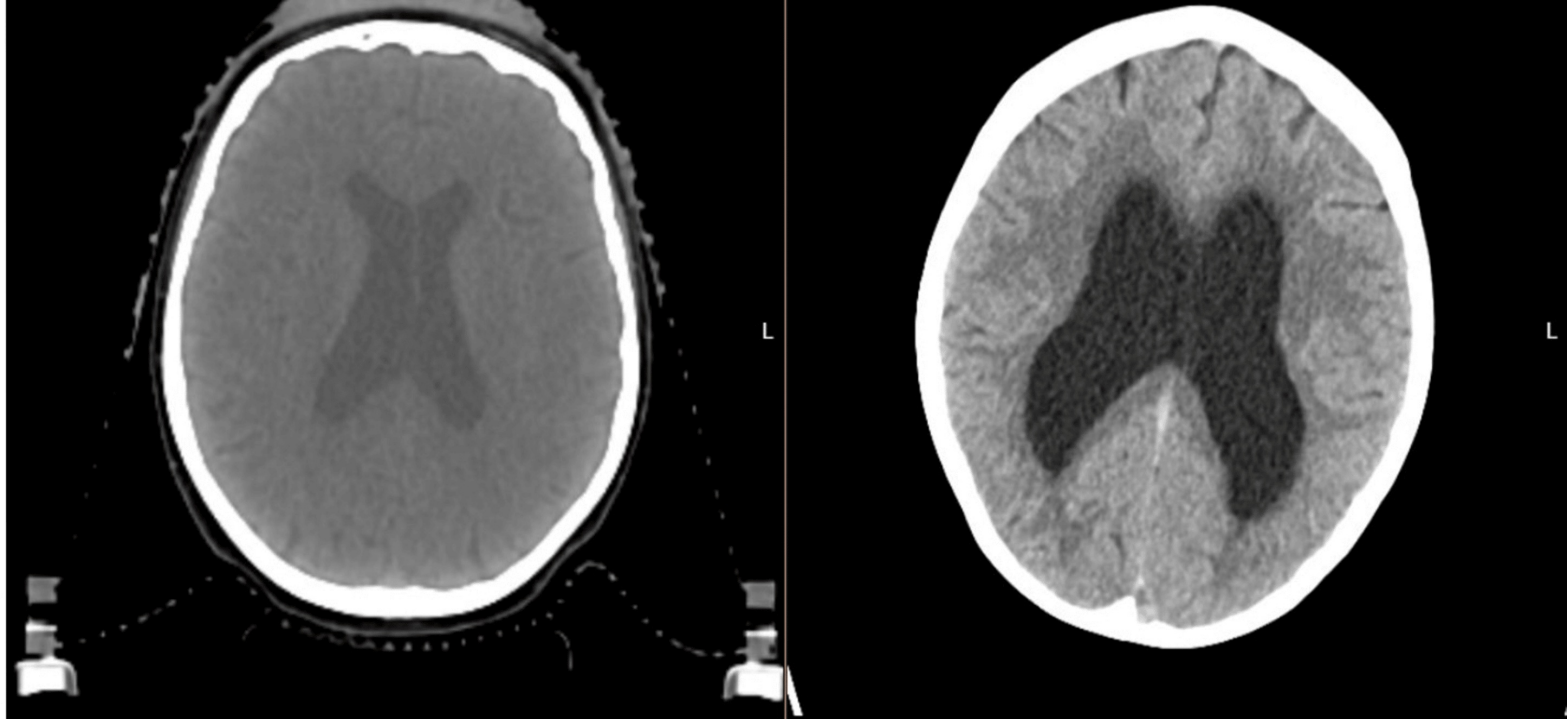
Radiotherapy planning CT before radiotherapy (left), and contrast CT 22 months after radiotherapy (right).
